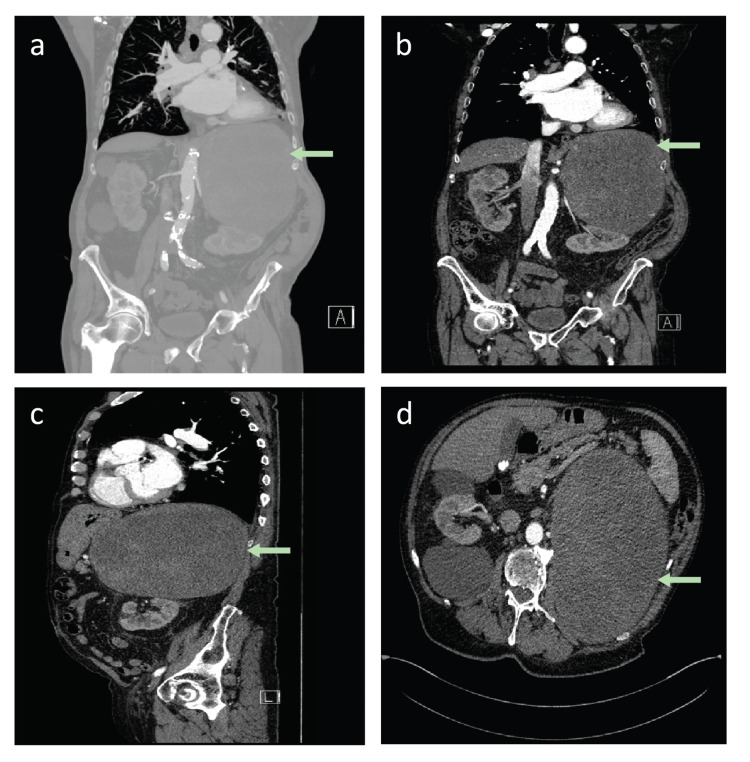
Computed tomography scan of the abdomen and pelvis in 2020. (a) Coronal view showing a large left suprarenal mass measuring 20.8 cm. (b) Coronal view showing the lesion was displacing the spleen, pancreas, left kidney, and in very close proximity to splenic renal vessels and the aorta. (c) Sagittal view. (d) Axial view.
